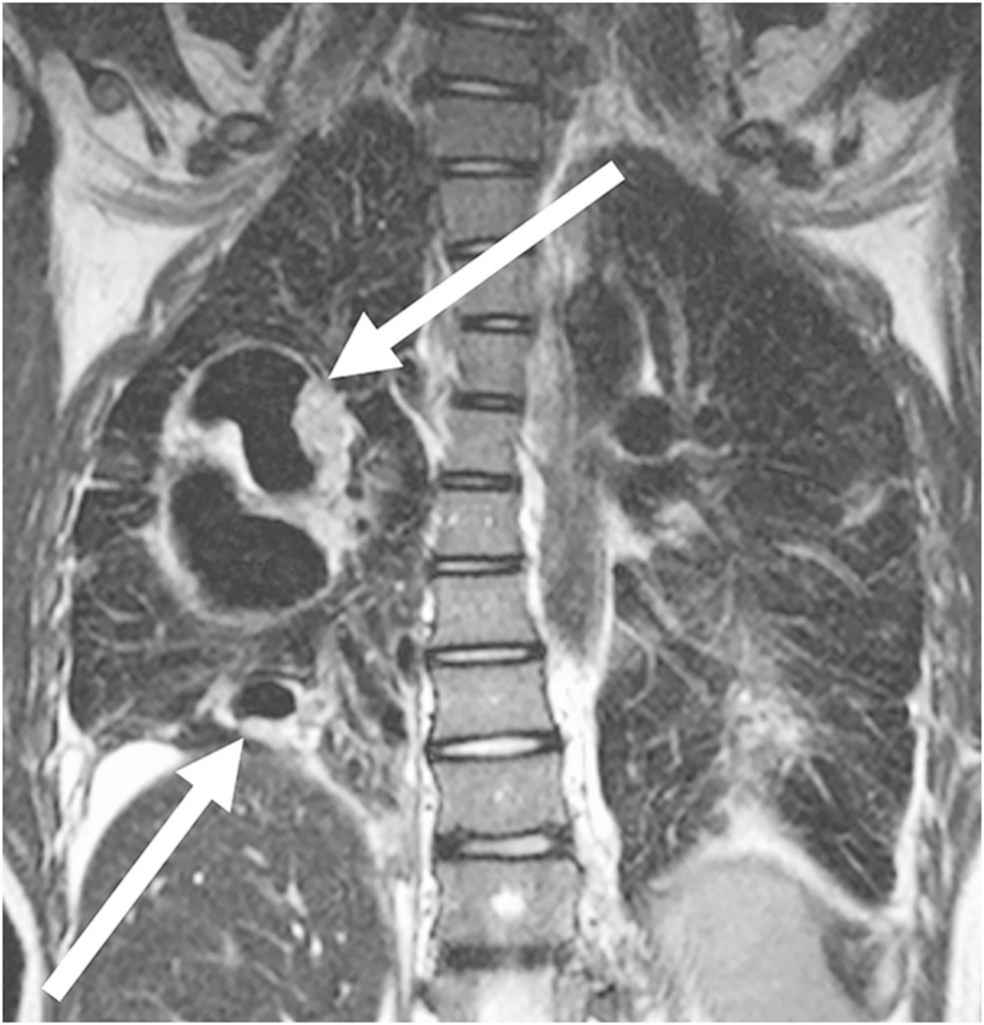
Rhabdomyosarcoma on lung MR in a 14-year-old girl. Coronal T2-weighted turbo spin-echo image (MR parameters in Table 2) shows partially cystic, partially necrotic metastases (*arrows*) from rhabdomyosarcoma
